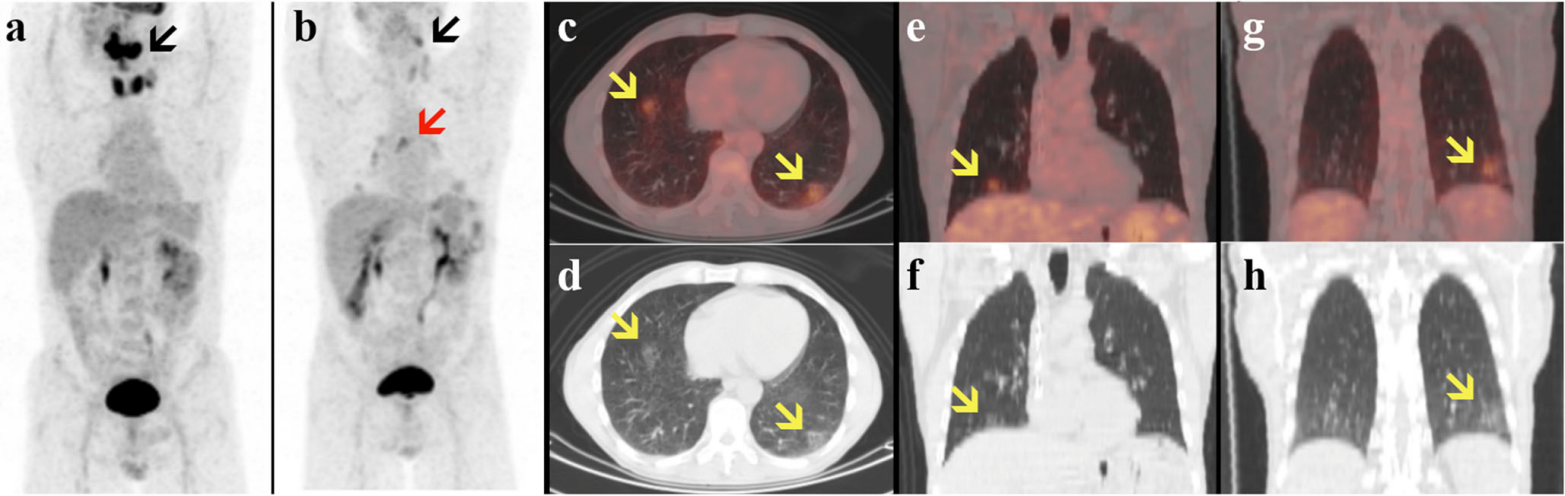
Previous (a) and current (b–l) [18F]FDG PET/CT of an asymptomatic 51-year-old man with a history of oral cavity squamous cell carcinoma. Maximum intensity projection (a–b), transaxial and coronal fused PET/CT (c, e, g), and CT (d, f, h) images of [18F]FDG uptake in the lungs
